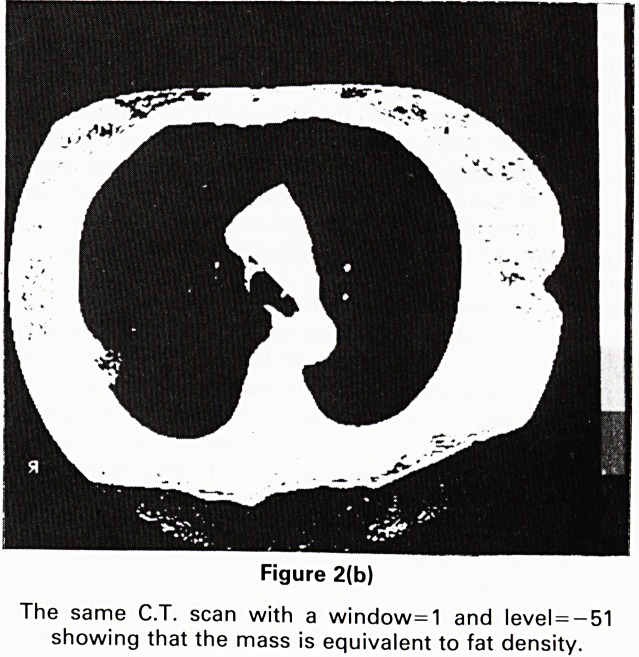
# The CT Diagnosis of Pleural Lipoma
